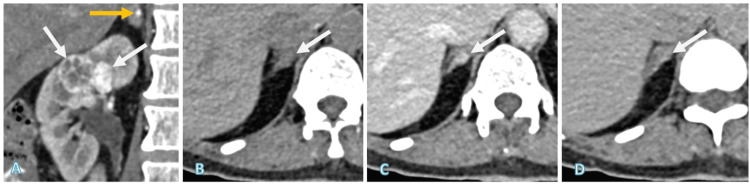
RCC metastasis – right adrenal: coronal CECT (A) shows an irregular hypervascular right renal mass (white arrows) with a synchronous right adrenal lesion (yellow arrow) showing similar hyperenhancement. Axial CECT shows a right adrenal nodule with high unenhanced attenuation (B) and avid enhancement (C), demonstrating rapid washout (D) mimicking adenoma; however, enhancement parallels known RCC, favoring metastasis. RCC = renal cell carcinoma; CECT = contrast-enhanced CT
